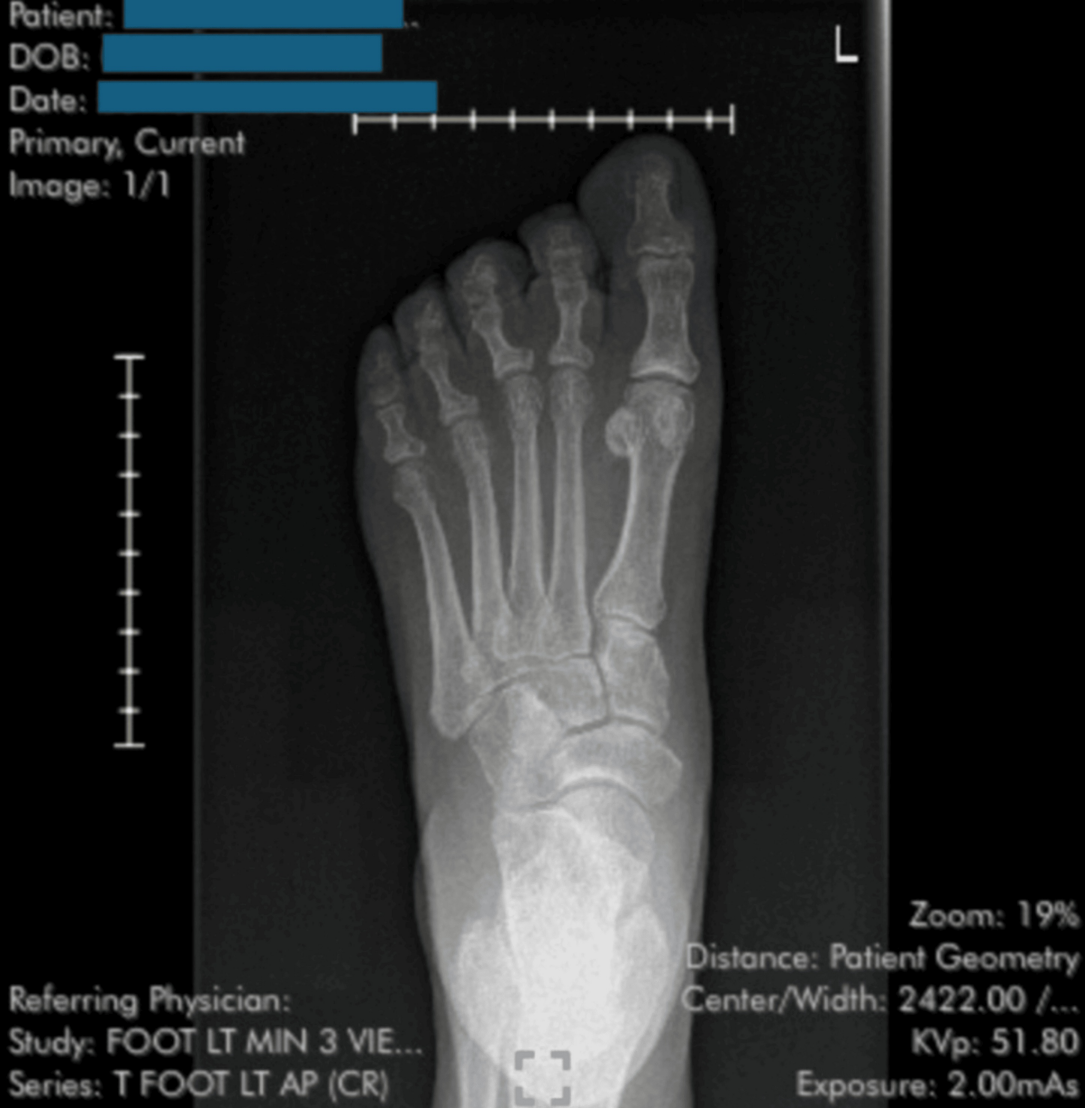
X-ray of the left foot showing no evidence of fracture or bony abnormality.
